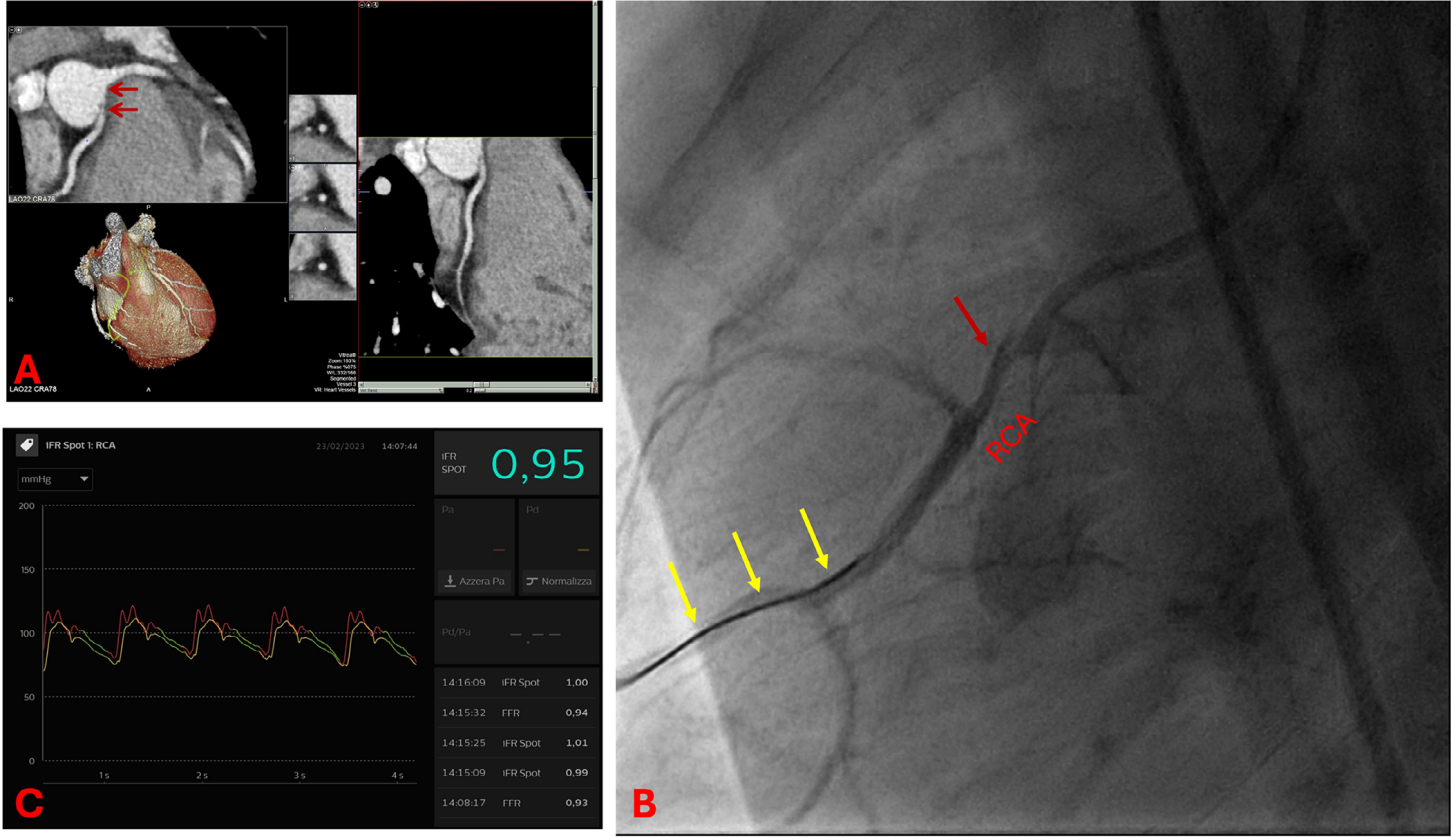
Anomalous origin of right coronary artery (RCA) from the left aortic sinus. (A) CT scan showed a long intramural course behind the right ventricular infundibulum. (B) Selective right coronary angiography showed an irregular shape of the proximal tract (red arrow). The tip of pressure guidewire (yellow arrows) was distal in the vessel. However, iFR plot (C) showed values within normal limits (> 0.93).
